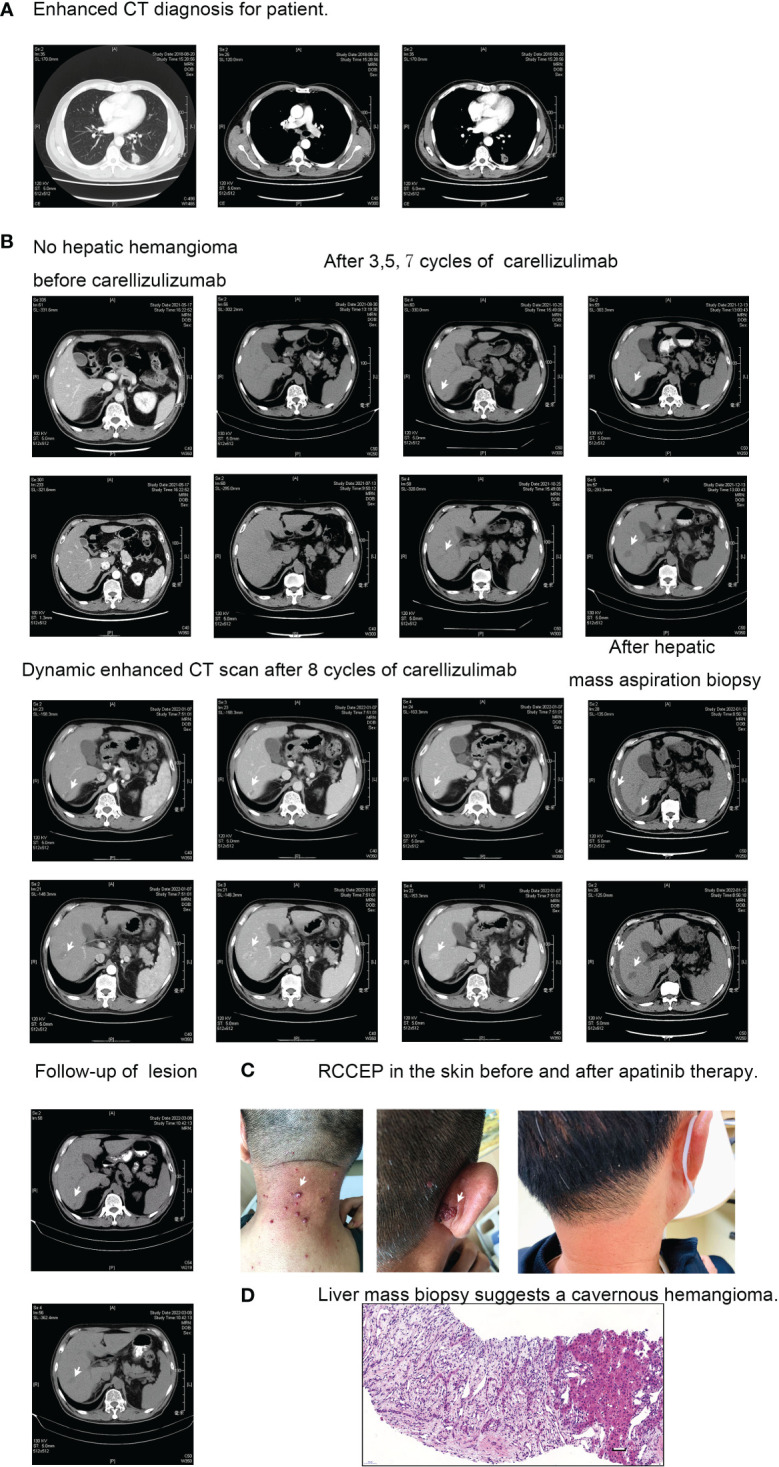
Diagnostic and treatment imaging. (A) Thoracic enhanced CT diagnosis. (B) Enhanced CT manifestation of hepatic cavernous hemangioma. (C) Skin reactions to RCCEP before and after apatinib treatment. (D) Pathological examination of hepatic cavernous hemangioma.
